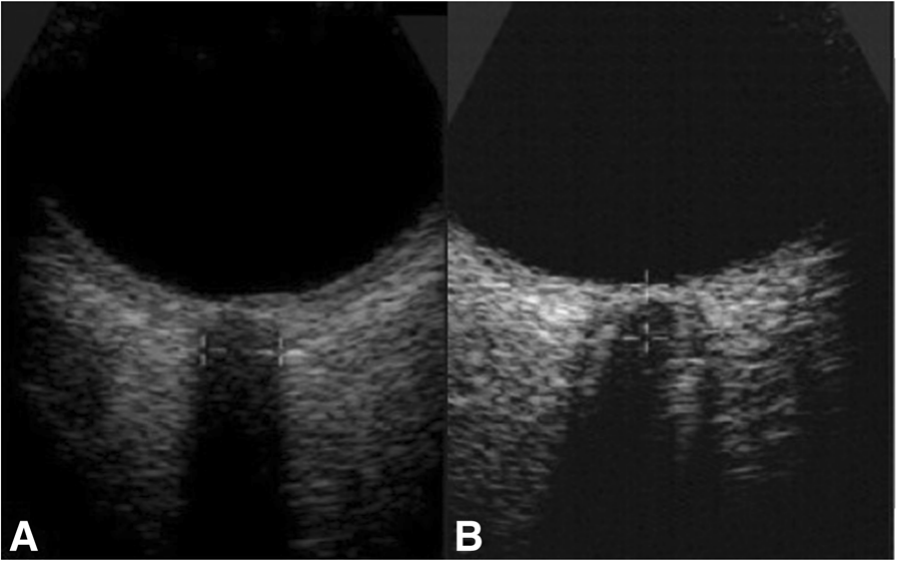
Ultrasound measurements of the axial plane of the right eye. a Optic nerve sheath of a patient with normal intracranial pressure. No subarachnoid space around the optic nerve. b Optic nerve sheath of a patient with high intracranial pressure. Significant subarachnoid space around the optic nerve
